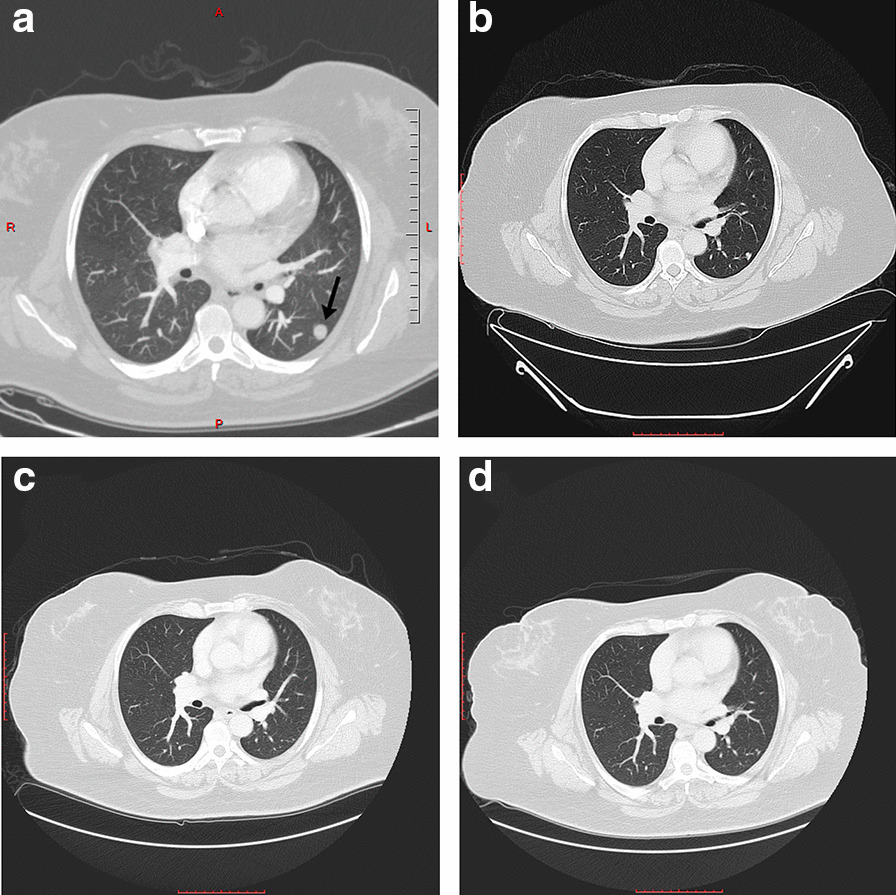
Transverse computed tomography image showing lung metastasis as pointed by the black arrow (a). Partial remission of the metastasized tumor was achieved after 8 months of letrozole 2.5 mg per day (b). Complete remission was observed after 18 months (c) and 2.5 years (d) with the same therapeutic regimen
